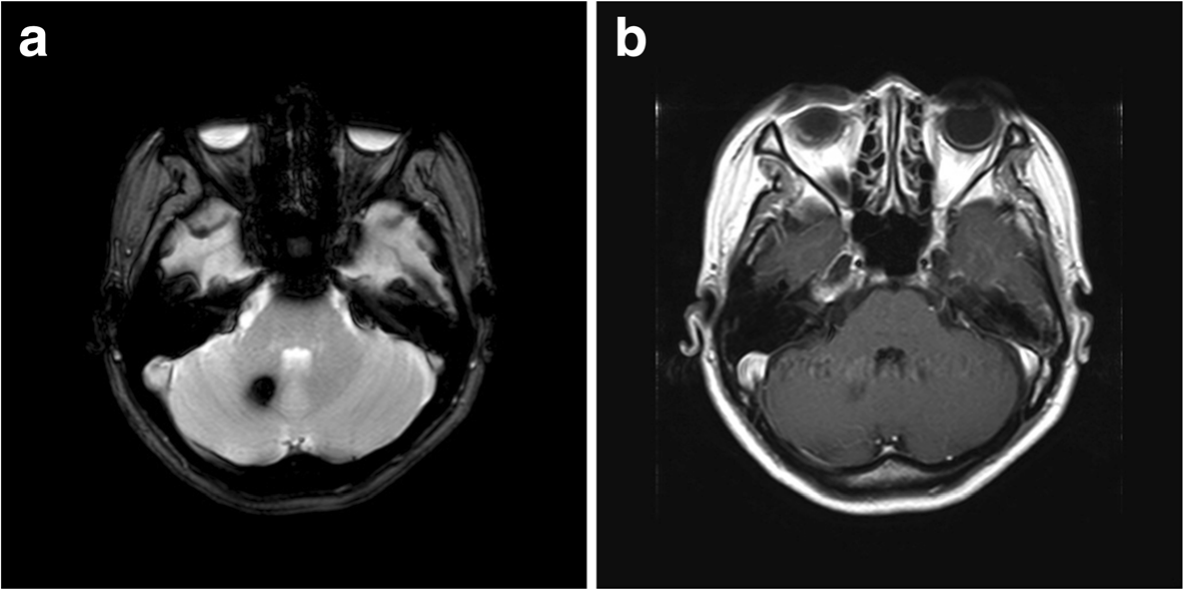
A 9.6-mm hemorrhagic lesion in the right cerebellar hemisphere. a T2-weighted gradient-echo cranial magnetic resonance image in the axial plane shows low signal intensity in the right cerebellar hemisphere. b Axial T1-weighted sequence shows no abnormally enhancing lesion following administration of intravenous gadolinium
